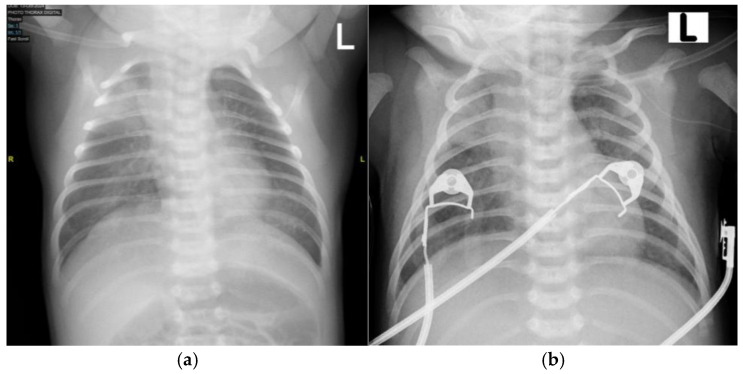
Anteroposterior (AP) chest X-ray showing (a) bilateral pneumonia on admission to the emergency department (ED) and (b) improvement in pneumonia on day 9 of hospitalization before extubation. “L”: an anatomical marker indicating the left side.
